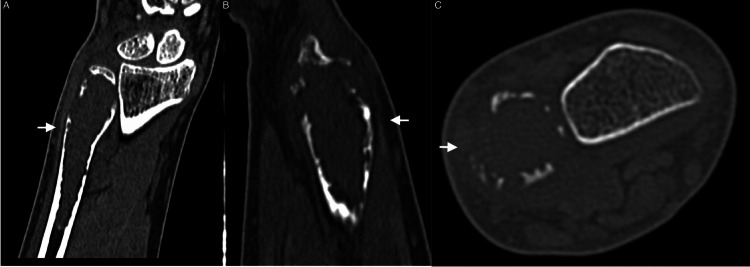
Coronal (A), sagittal (B), and axial (C) CT scan of the left distal ulna showing a lytic lesion in the ulnar metaphysis, extending into the epiphysis and diaphysis and measuring 1.5 x 1.6 x 6.4 cm.
